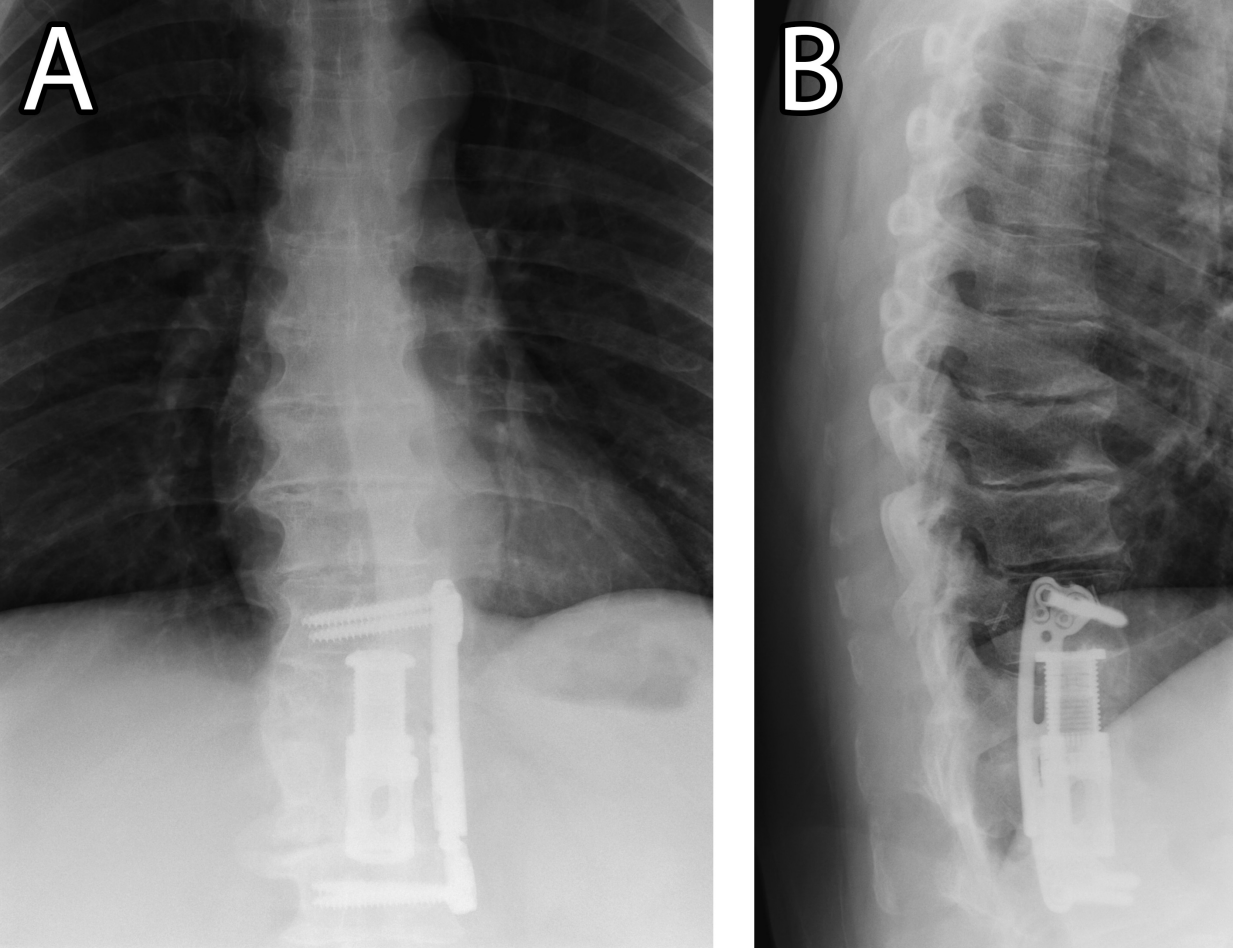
Postoperative lumbar X-rays A) Postoperative anteroposterior and B) lateral lumbar X-rays demonstrating the final spinal alignment, position of instrumentation, and resolution of infection one-year postoperatively.
